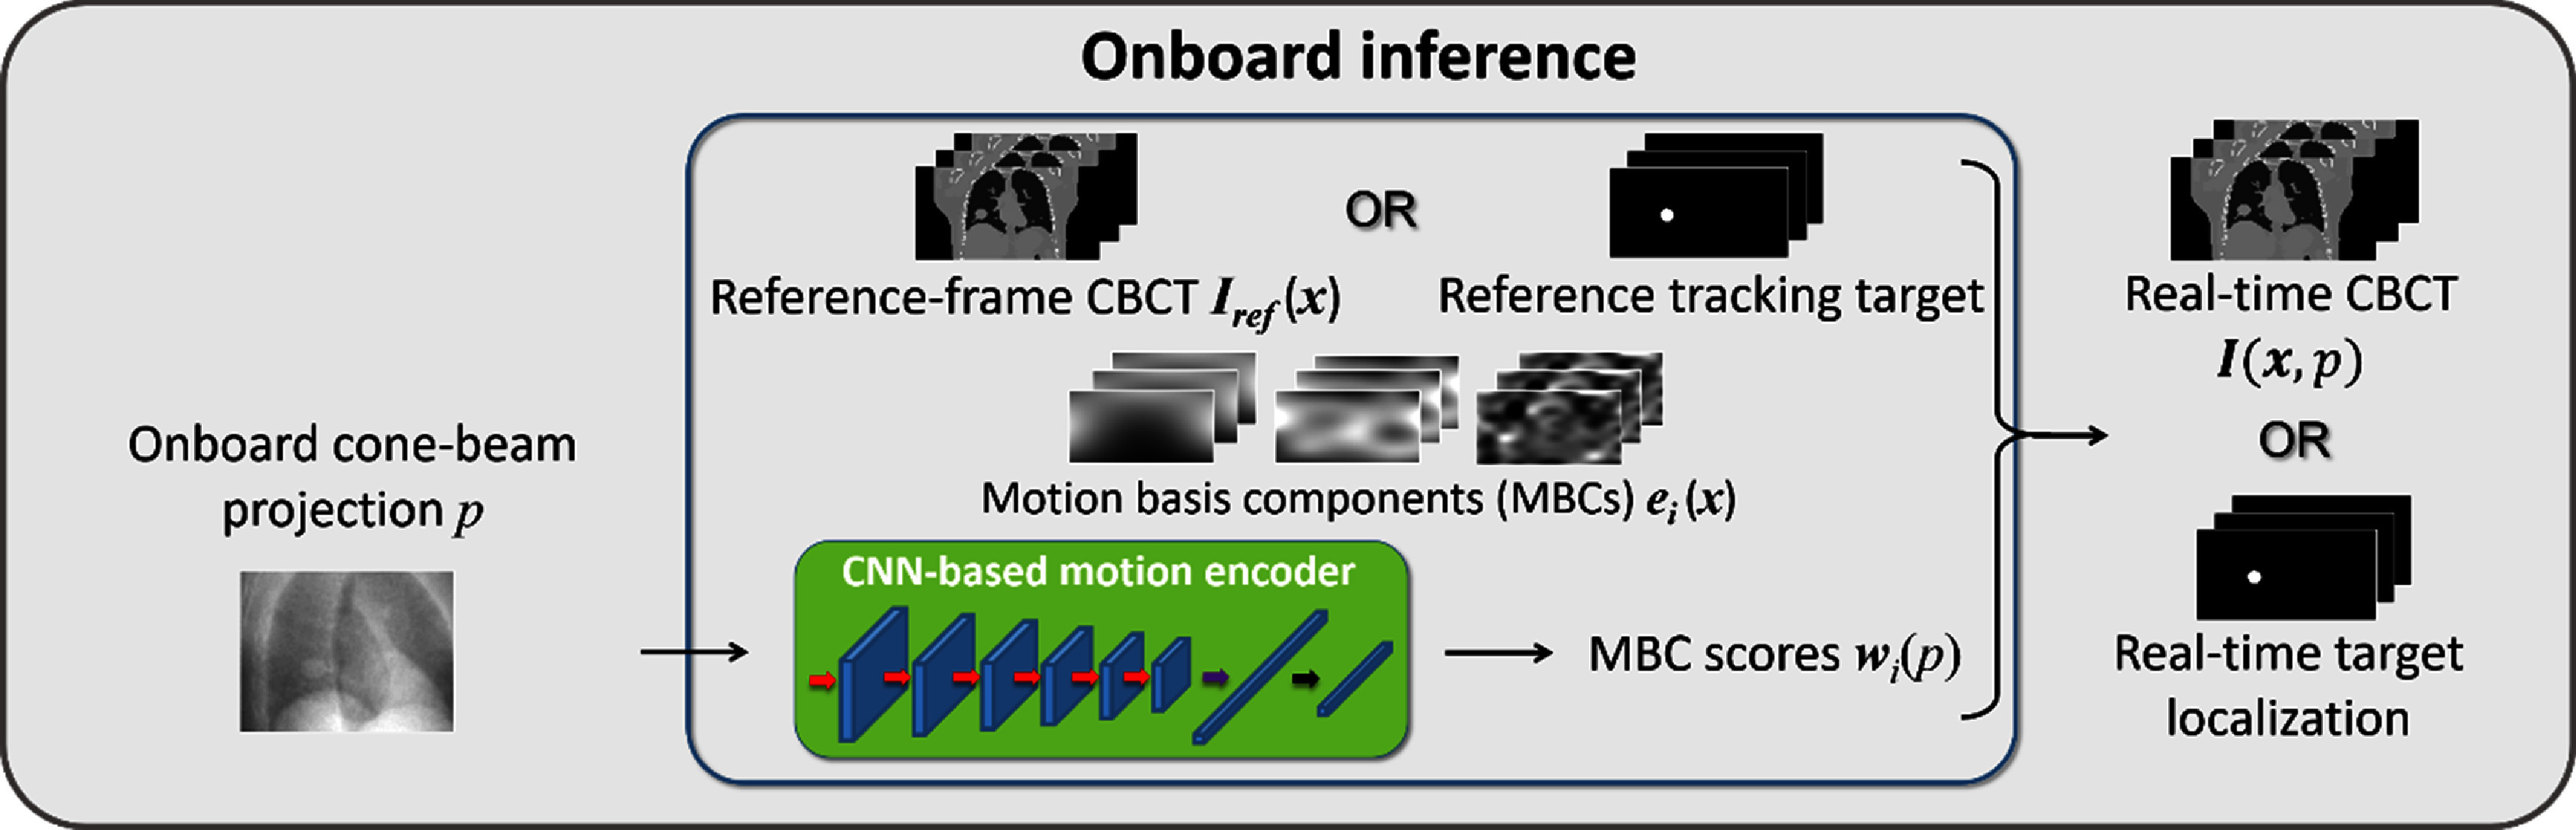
Onboard inference stage for real-time CBCT imaging and target localization. During the onboard inference stage, the CNN-based motion encoder takes an onboard projection p at an arbitrary angle as input, and estimates the MBC scores ${{\boldsymbol{w}}_i}\left( p \right)$ to derive real-time CBCT ${\boldsymbol{I}}\left( {{\boldsymbol{x}},{\text{ }}p} \right)$ or 3D target.
